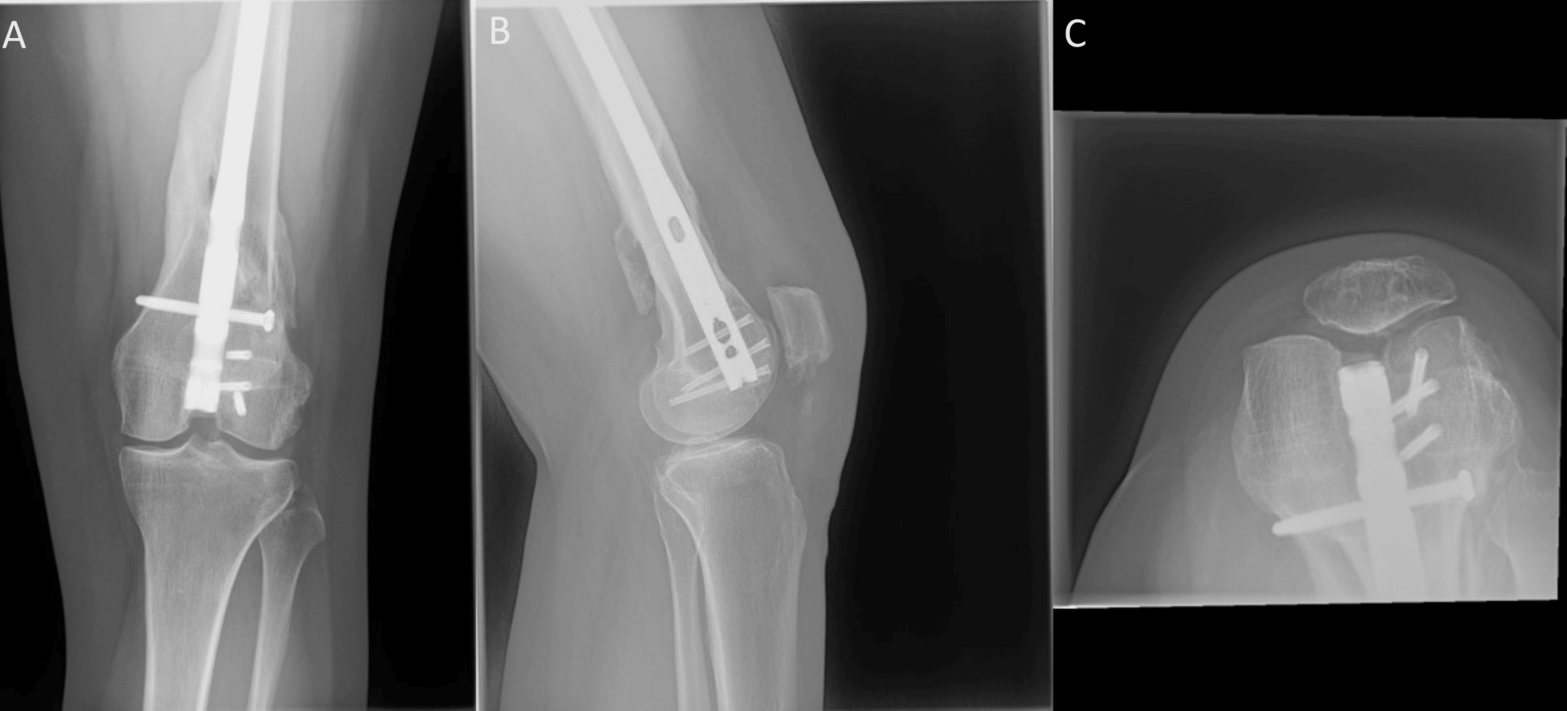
Six months postoperative osteochondral allograft transplant: (A) AP, (B) lateral, and (C) sunrise views of the left knee revealing no interval change since the previous radiographs. AP: Anteroposterior.
